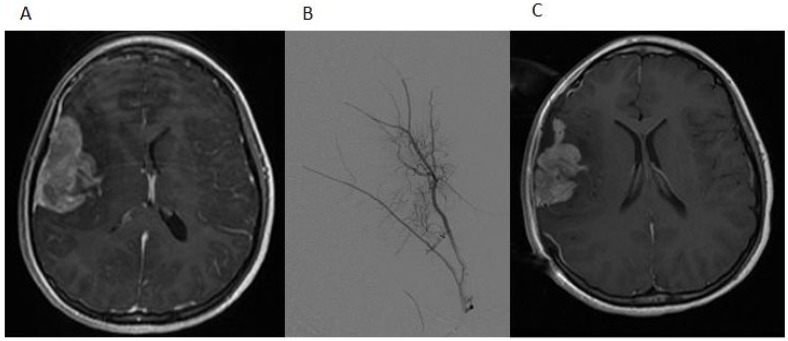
(A) The post-contrast T1-weighted MRI image shows a predominantly heterogeneously enhancing mass extending into the right Sylvian fissure with probable invasion of the adjacent insular cortex; (B) a select image from the tumor embolization demonstrates a dramatically hypervascular tumor supplied by branches of the right middle meningeal artery that was successfully embolized with polyvinyl alcohol particles; (C) the postoperative T1-weighted MRI image with contrast demonstrates partial resection of the tumor.
